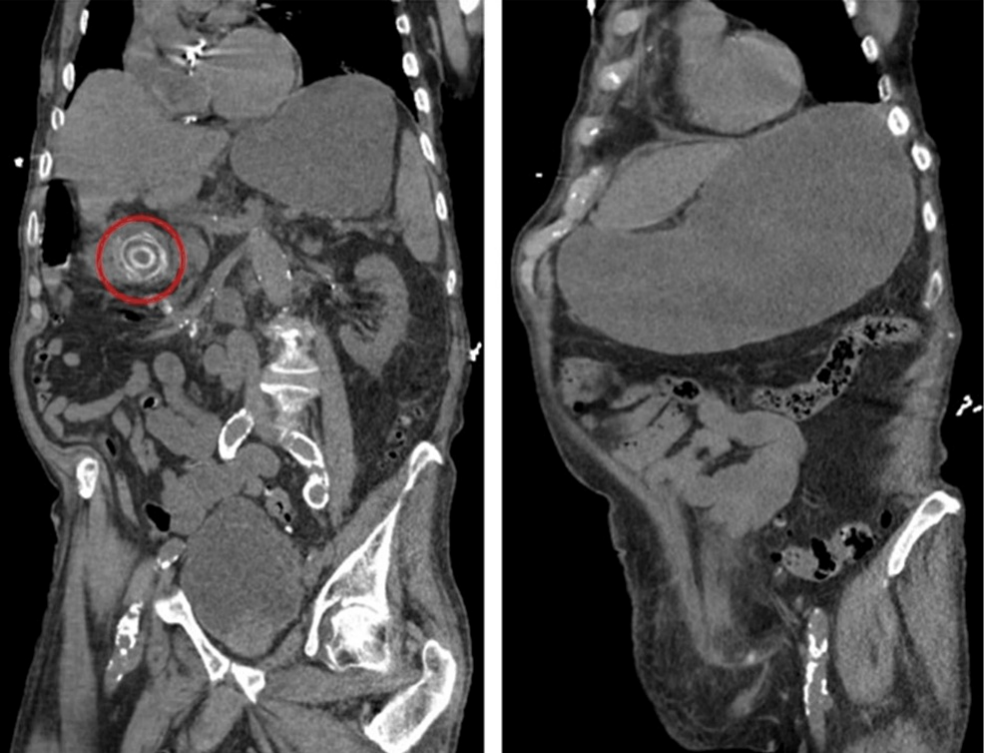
Initial computed tomography of the abdomen revealed (A) a large gallstone in the first portion of the duodenum causing (B) gastric outlet obstruction.
